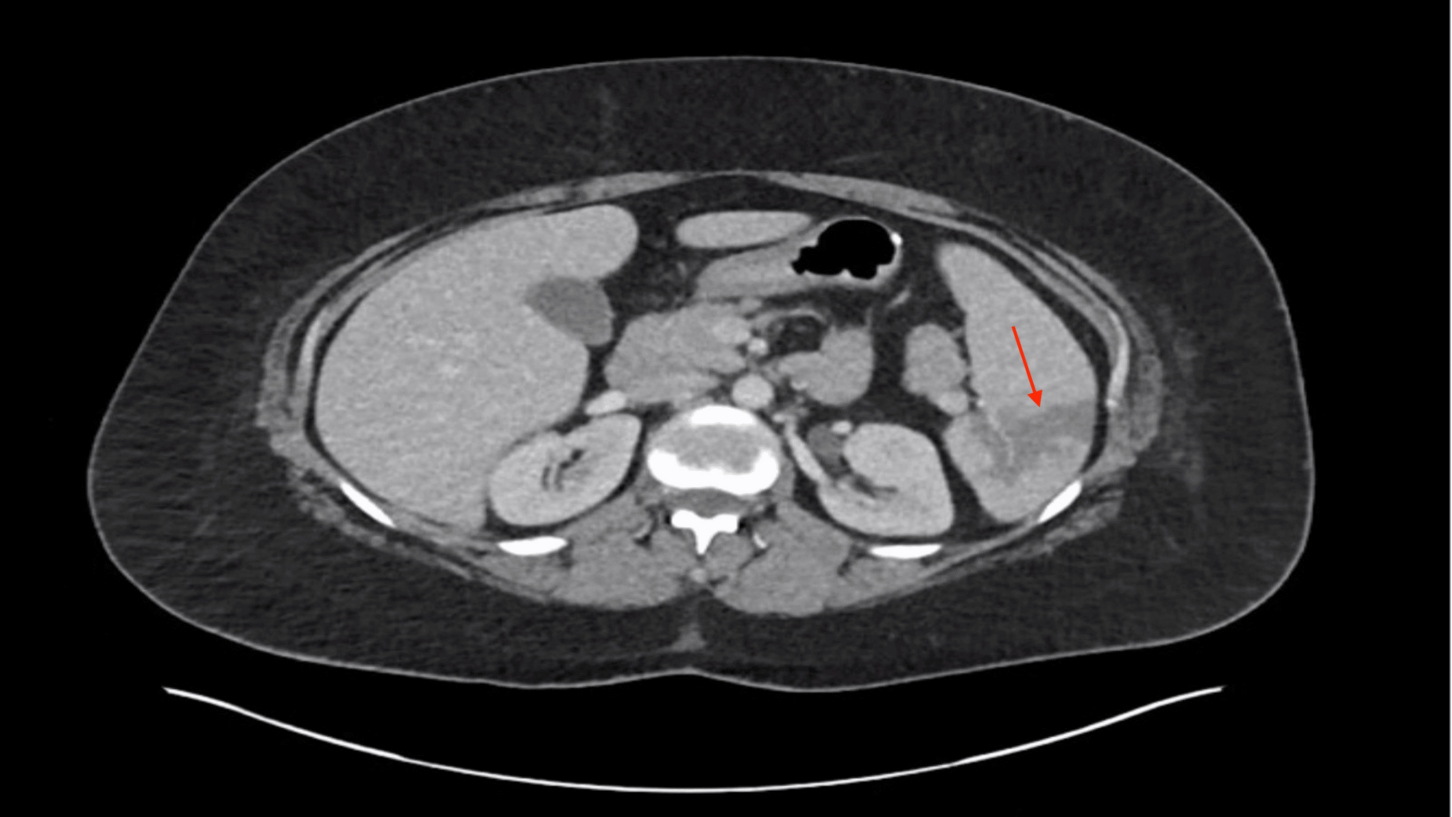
A CT scan of the abdomen showing a splenic infarct (red arrow).
